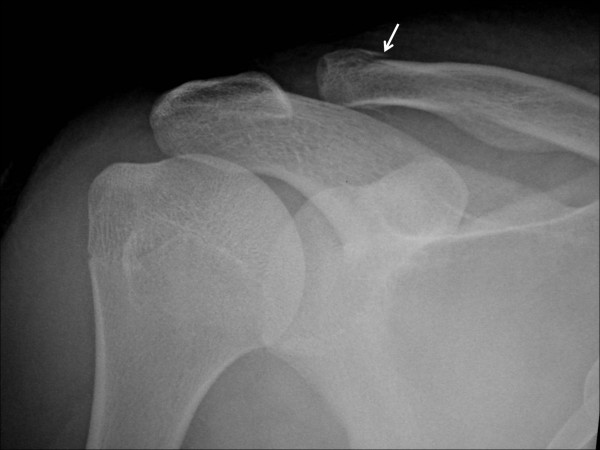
Roentgenogram at the time of injury. A non-displaced fracture (white arrow) at the distal end of the right clavicle was observed.
